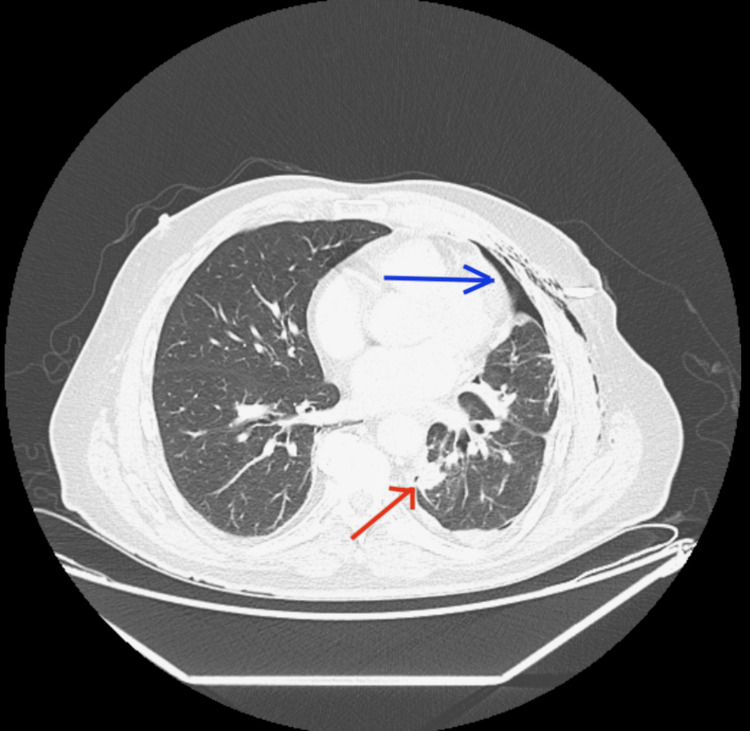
CT chest with contrast showing pleural-based masses in the left lung (red arrow) and small pneumothorax (blue arrow).
